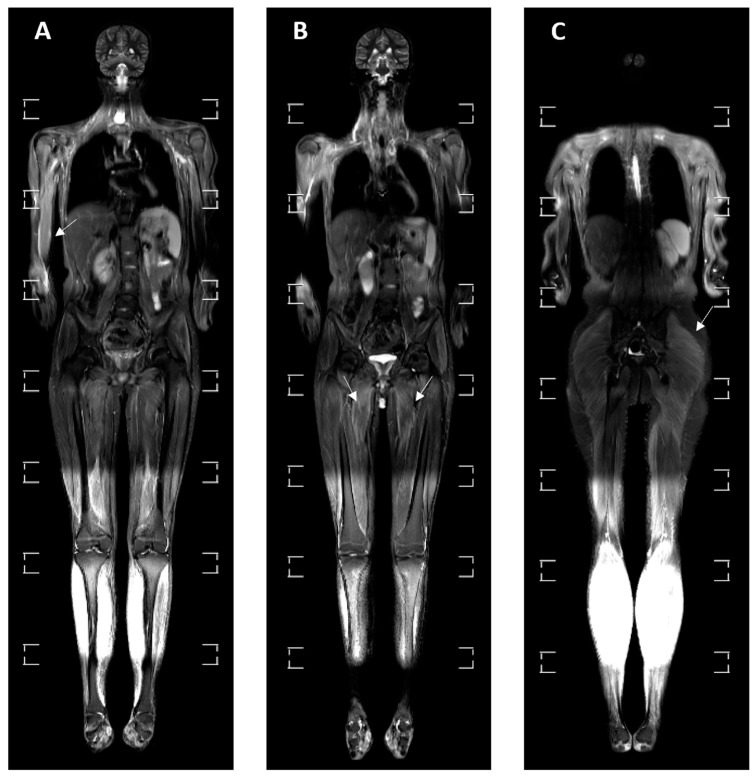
Three consecutive cross-sections (A–C) of the whole-body MRI in the STIR sequence of a 16-year-old male patient with JIIM and a history of muscle weakness. (A) An elevation of signal in the muscles of the right hand and forearm. (B) An elevation of signal in the adductor muscles of both thighs is visible, pointed by white arrows. (C) An elevation of signal in the left gluteus maximus muscle is notable, pointed by arrow.
